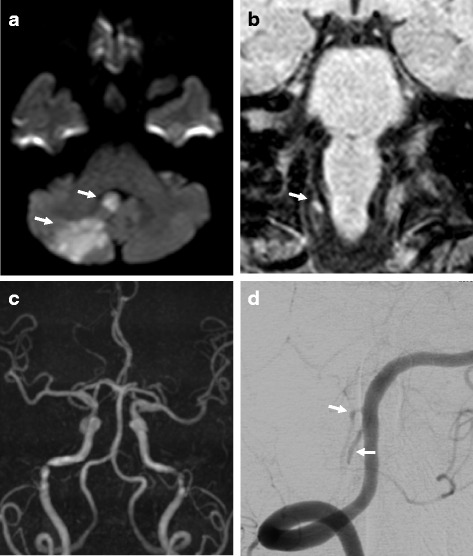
Imaging findings in Case 1. MRI on admission showed high-intensity signal areas in the cerebellar vermis and the right cerebellar hemisphere on DWI (a, arrow) and a high-intensity signal area beside the right VA on T1-VISTA (b, arrow). The right PICA was not detected on MRA (c). A right vertebral angiogram at 9 days after admission showed the “pearl and string sign” at the proximal portion of the right PICA (d, arrow)
